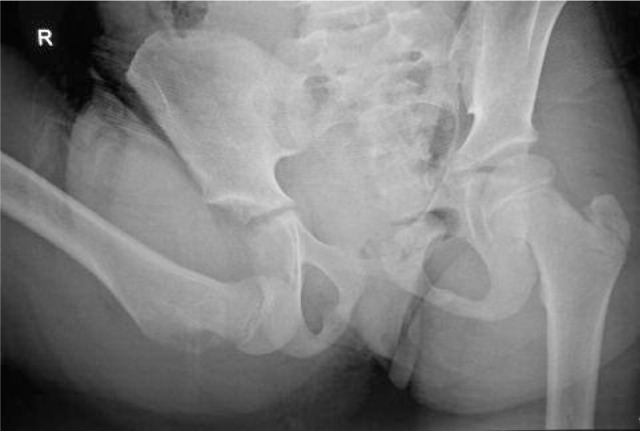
Plain radiograph of the child showing anterior obturator dislocation of right hip.
